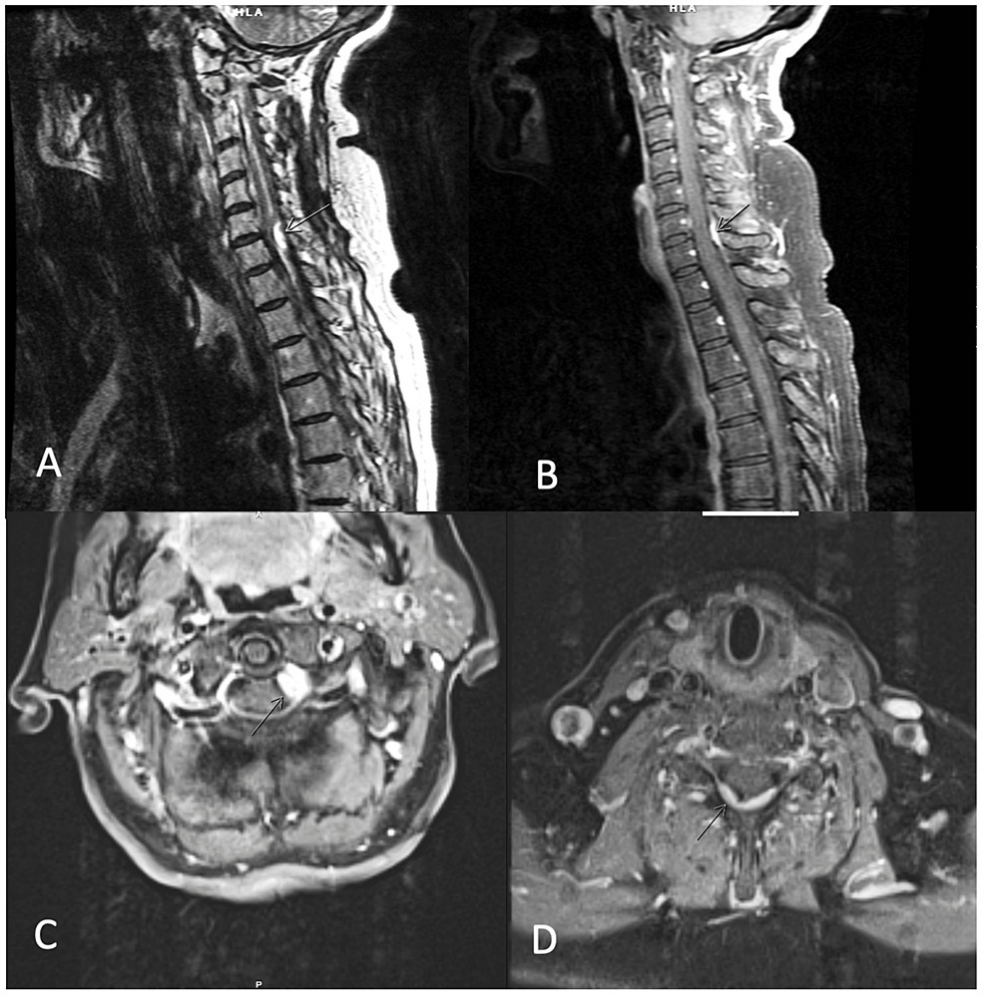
Spinal metastases secondary to esthesioneuroblastoma MRI scan demonstrating dural enhancement at T5 level; A*:* T2 scan with contrast, B*:* T1 scan with contrast
